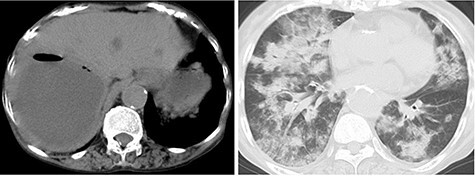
Abdominal and chest CT at admission showing a cyst containing air-fluid levels in the right hepatic lobe measuring 106 mm × 103 mm, and bilateral consolidation in the lungs.
